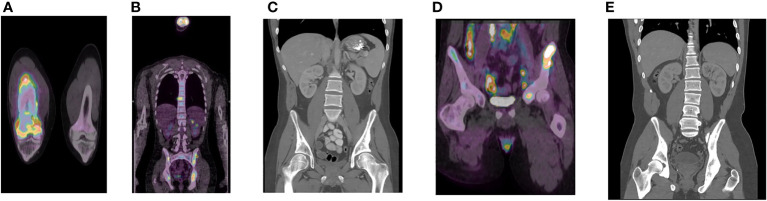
Diagnostic imaging of NPC metastasis. PET scan demonstrating bony lesions in the right femur (A) and multiple iliac bony lesions and metastatic adenopathy in the abdomen, retroperitoneum, and chest (B) prior to gemcitabine/cisplatin (G/C) chemotherapy. CT scan after 4 cycles of G/C chemotherapy demonstrating resolution of metastatic adenopathy (C). PET scan after 8 cycles of G/C chemotherapy demonstrating cancer progression in the ilium, retroperitoneum, and pelvis (D). Latest CT scan to date (22 months post-pembrolizumab) demonstrating stable healed bony metastasis (E).
